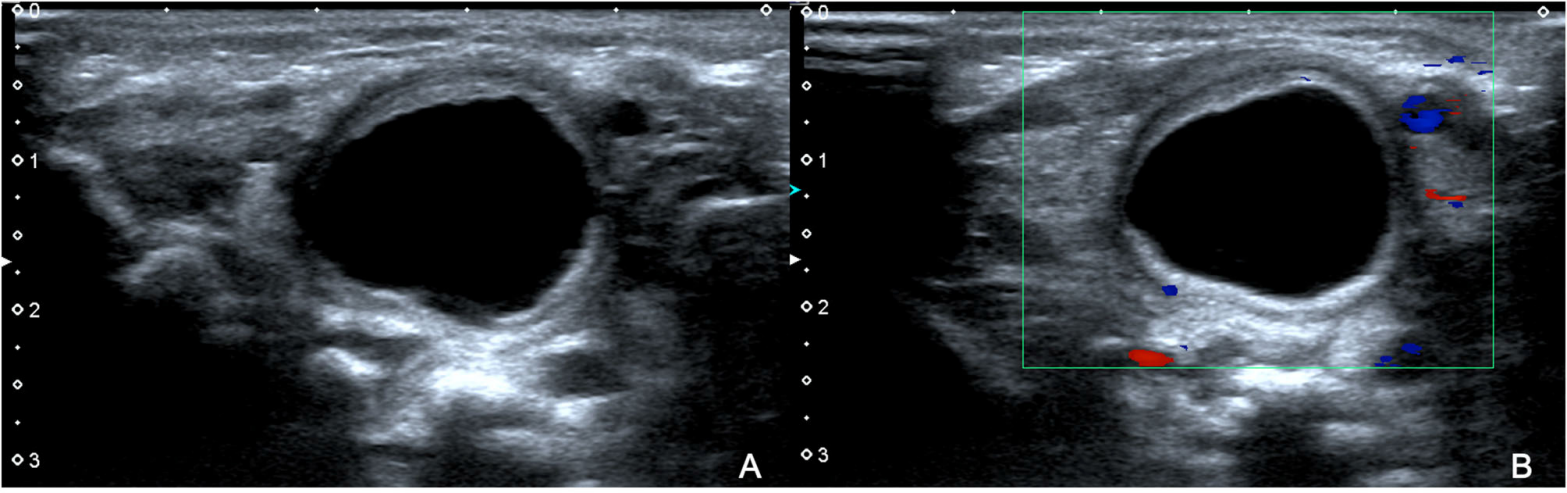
Ultrasound revealed a cyst on the right side of the neck in the patient at the age of 2 days. The cyst was 2.2 × 1.8 × 2.1 cm in size, and had a wall thickness of 0.2 cm (A). Color Doppler flow imaging (CDFI) showed no obvious blood flow signal on the edge and wall of the capsule (B).
